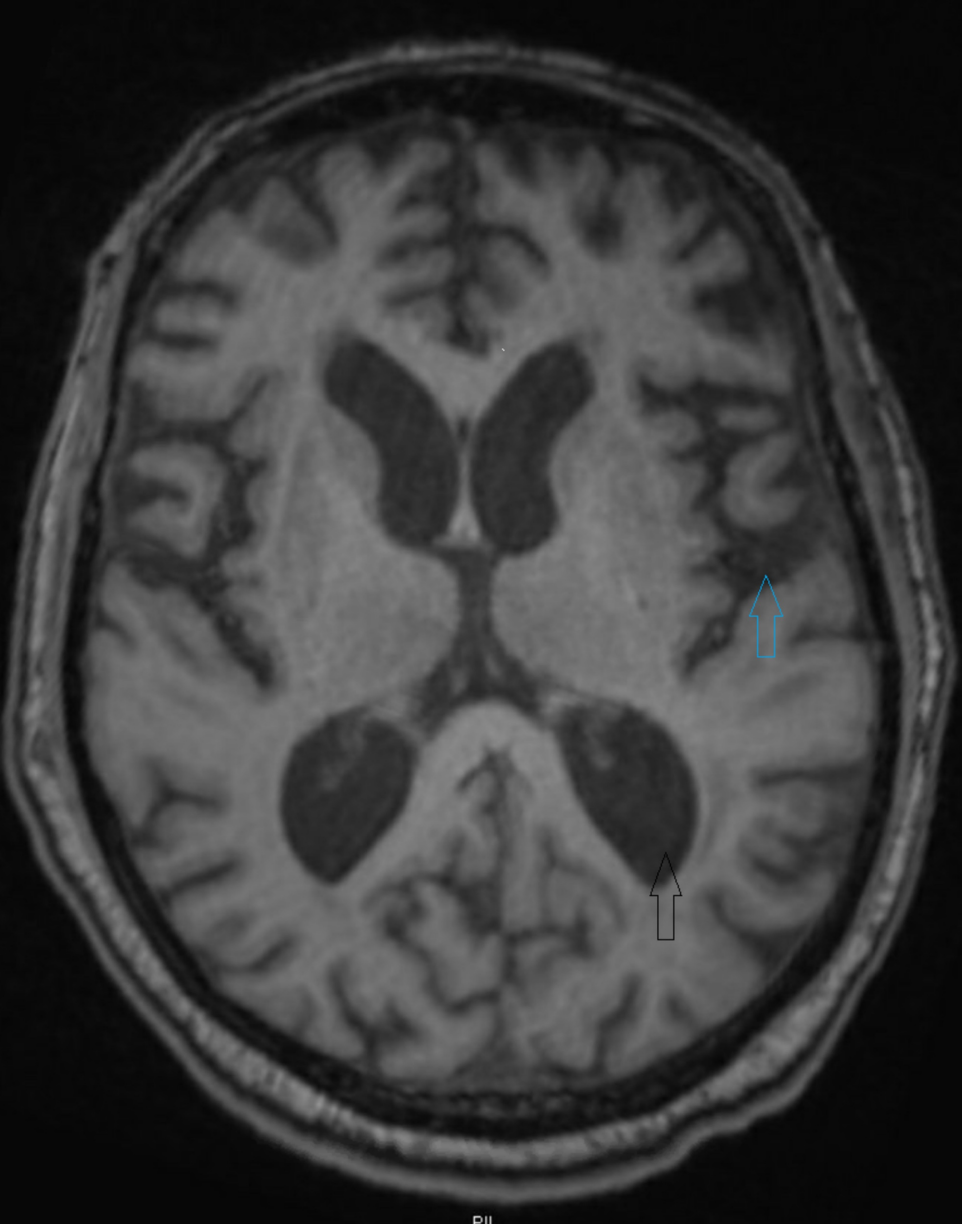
MRI brain without contrast showing age-related mild volume loss(upper blue arrow) and prominent ventricles(lower black arrow).
